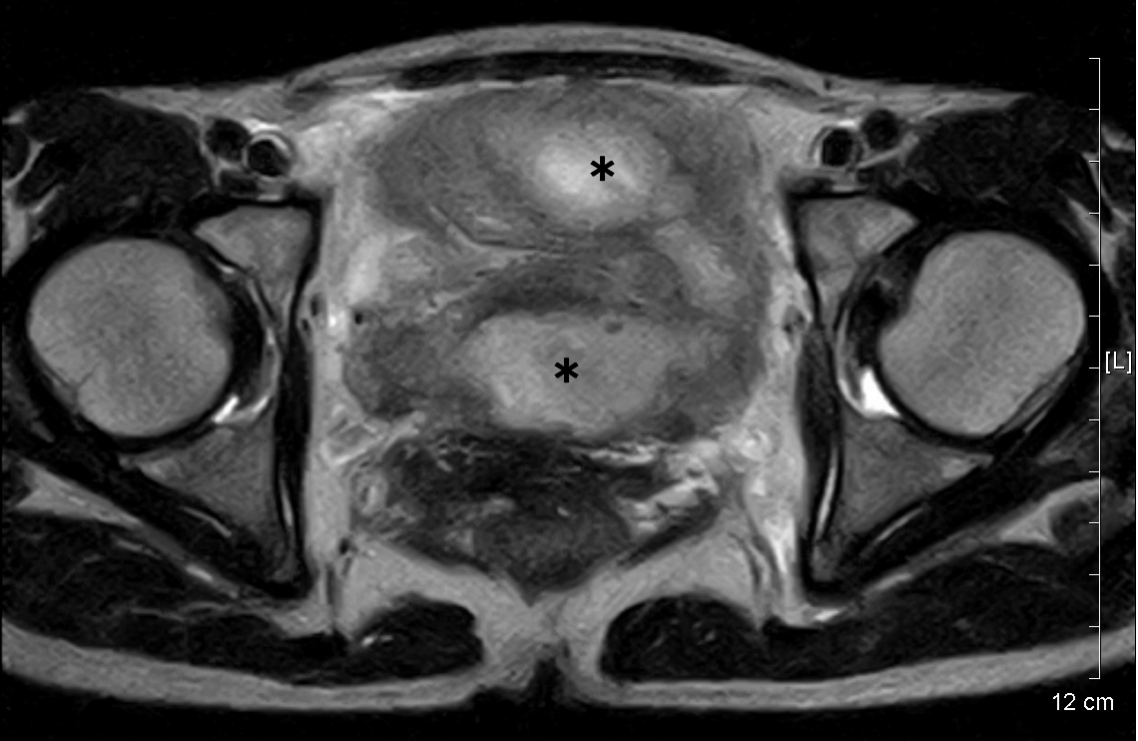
An oblique magnetic resonance T2-weighted image disclosed a horseshoe-shaped abscess, 8 × 7 cm in size (indicated by asterisks). The abscess wall is of intermediate to slightly high intensity whereas the fluid inside exhibits high intensity signal.
